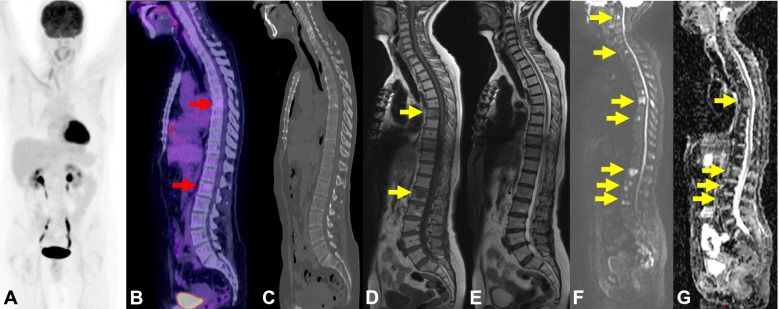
FDG PET-CT and WB MRI in lobular breast cancer. 62-year-old female with a history of treated lobular breast cancer with no history of metastatic disease presented with back pain. FDG PET-CT MIP (A) showed no significant or high grade pathological activity. The fused PET-CT images (B) showed minimal activity in a few bone lesions (red arrows) although the lesions were barely appreciated on the low-dose CT component (C). These were indeterminate but suspicious in this context. WBMRI was performed for further evaluation which showed a greater number of bone lesions (yellow arrows) better seen on Sagittal T1-weighted images (D) compared with the Sagittal T2-weighted images (E). The bone lesions demonstrate restricted diffusion with high signal on b900 DWI images (F) and low signal on ADC map (G) in keeping with bone metastases
